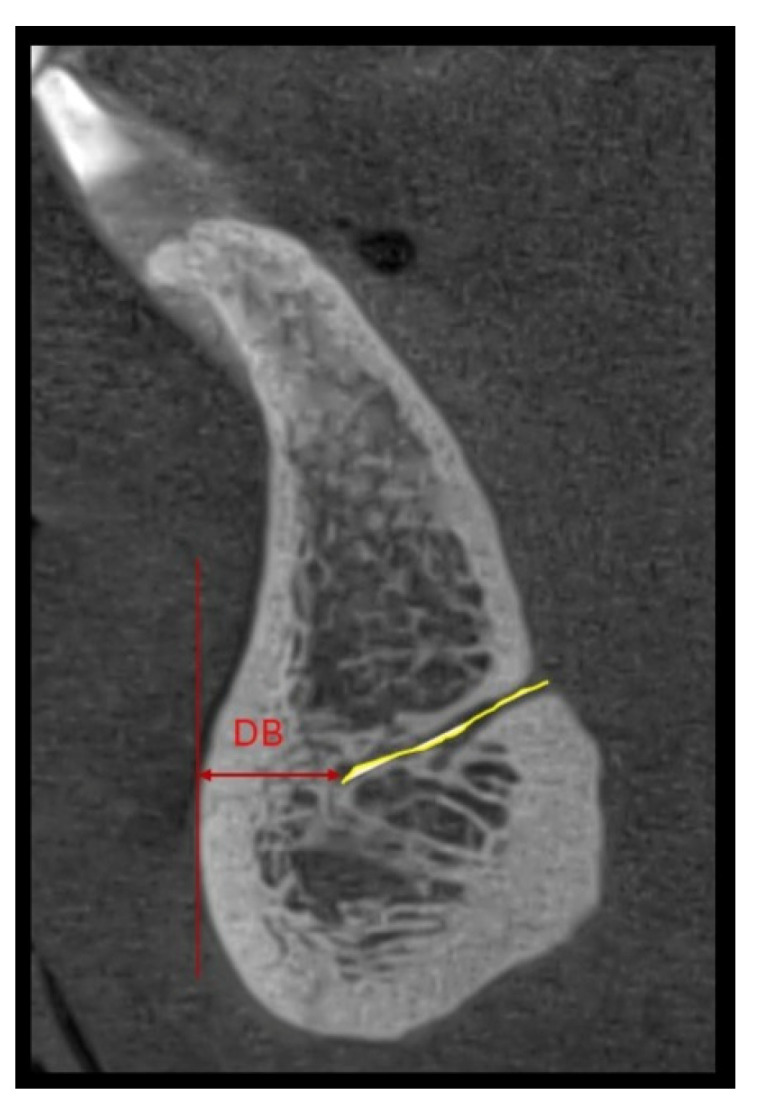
CBCT sagittal cut showing the deepest buccal distance of the MLF (DB). Yellow line—lingual canal.
